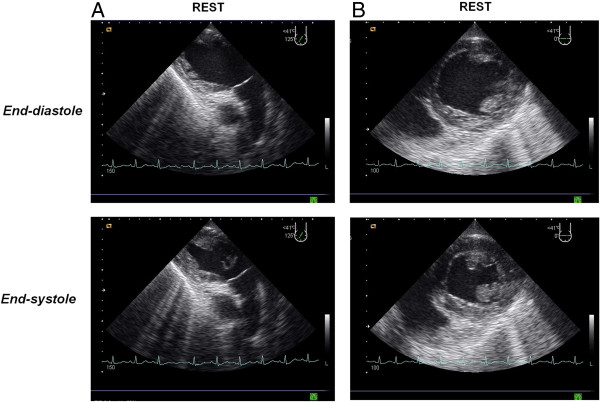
Donor #6 of Table 1. Two-dimensional transesophageal echocardiogram recorded immediately after “explosive” subarachnoid hemorrhage accompanied by extreme systolic hypertensive response with systolic blood pressure ≈ 300 mmHg and acute pulmonary edema. The donor had discrete left ventricular dysfunction with hypokinesia involving the inferior and lateral LV walls [see Additional file 3].
